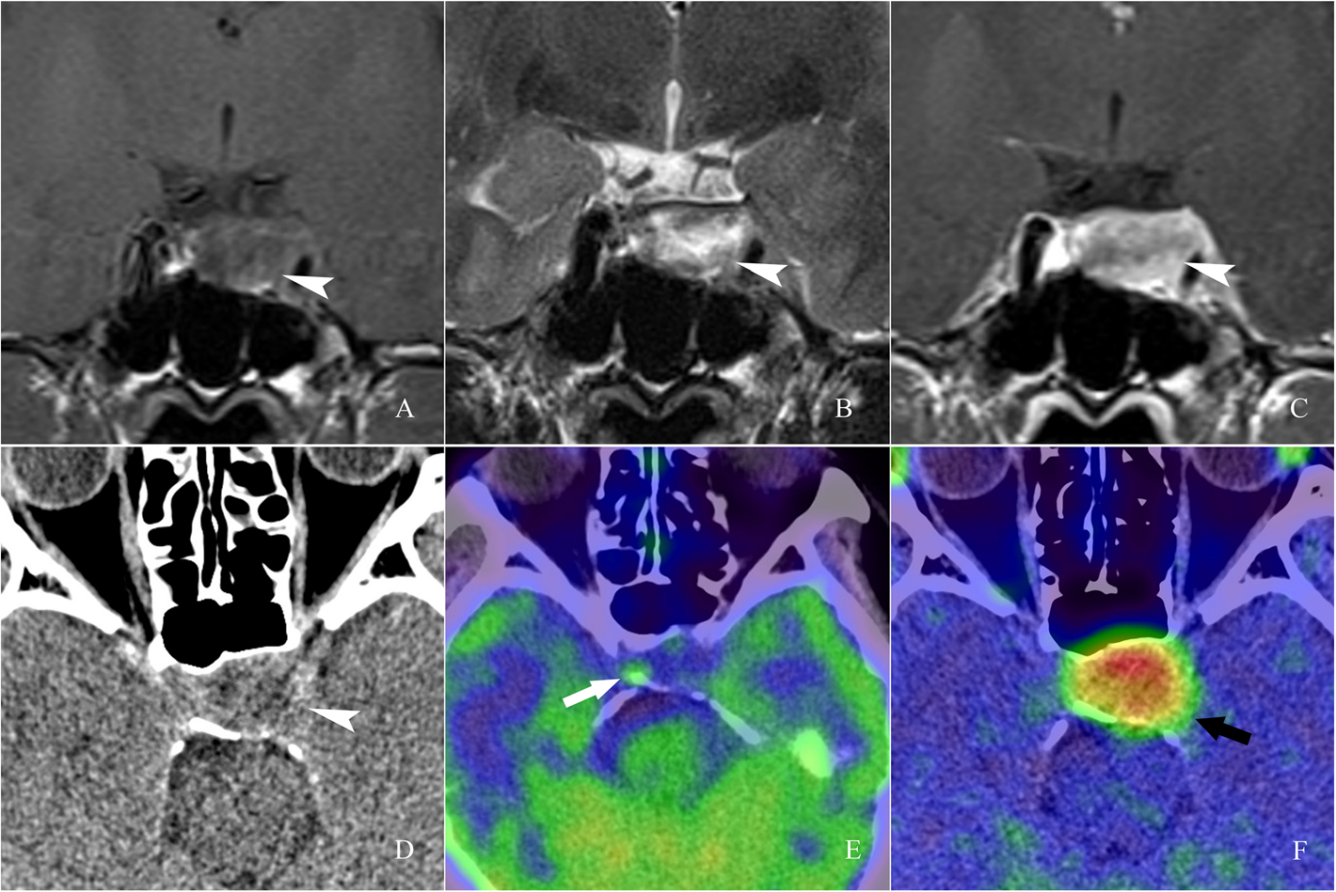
Images of a 37-year-old woman with recurrent prolactin (PRL)-secreting pituitary adenoma. T1-weighted coronal image, T2-weighted coronal image and T1-weighted contrast-enhanced coronal image showed an adenoma in the sella (A, B, C arrowhead). On ^13^N-ammonia PET/CT, a moderate-metabolic region in the right part of the sella was observed, revealing the pituitary gland remnant with hypopituitarism (E, white arrow). On ^11^C-MET PET/CT, an extreme hypermetabolic region in the the sella was observed, which was consistent with the CT and MRI images (F, black arrow)
